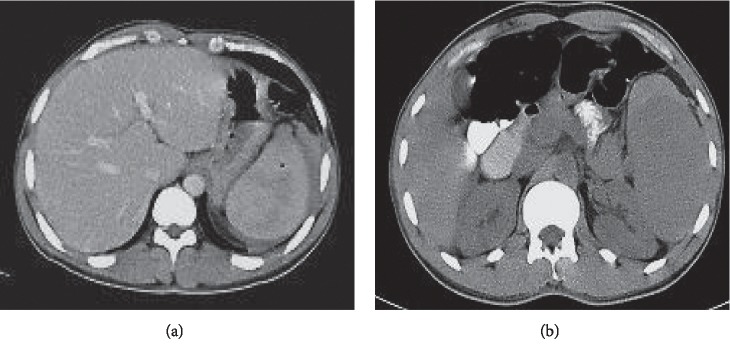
(a, b) Contrast-enhanced abdominal CT demonstrating subcapsular haematoma with grade II splenic rupture.
